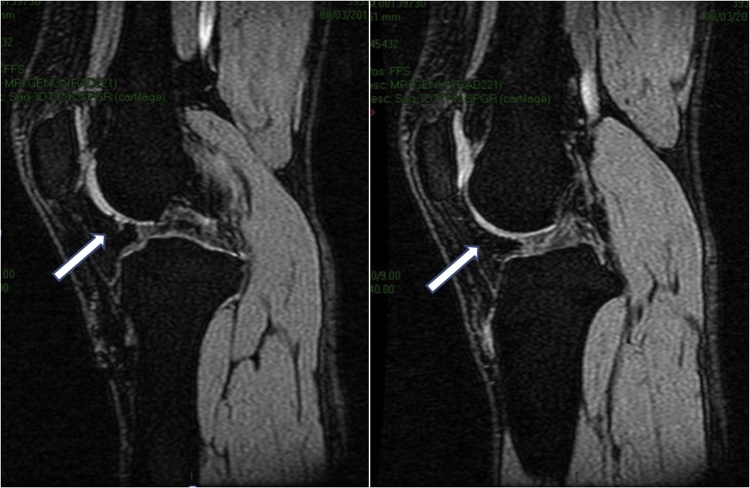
MRI FSE cor T2-weighted signal in different slice. Left image showing cartilage defect (pointed by the arrow). Right image showing cartilage growth was found in the defect (pointed by the arrow).
